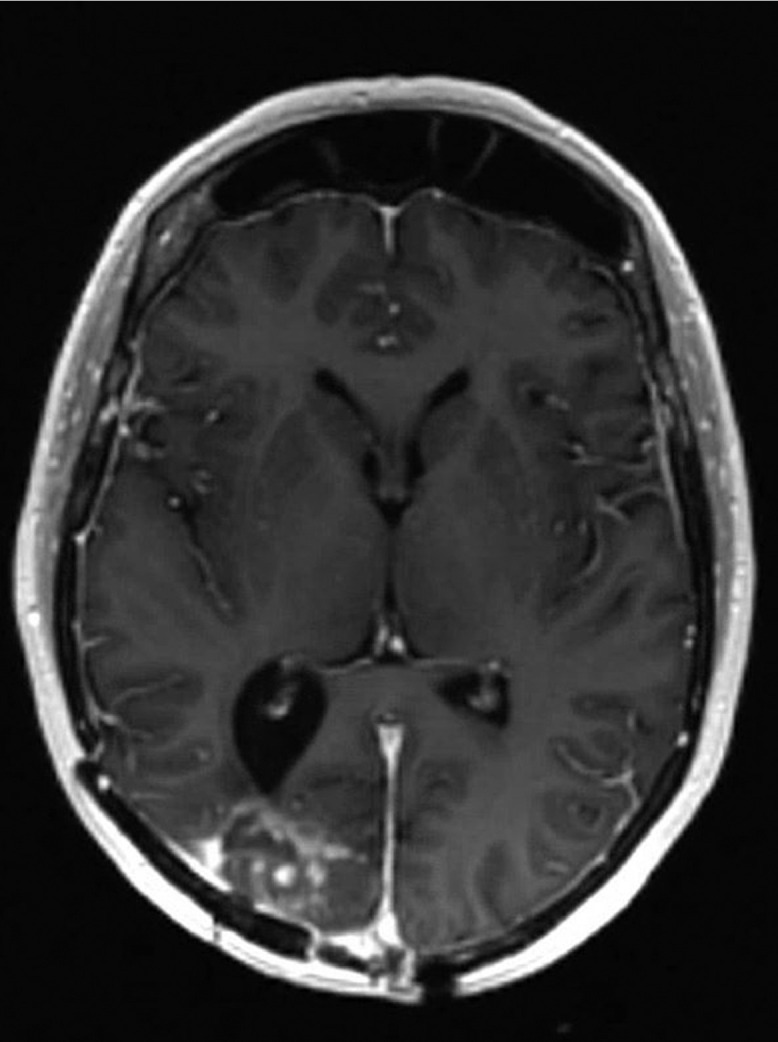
Magnetic resonance image of the brain in April 2019; local recurrence predominantly in the right occipital lobe.
